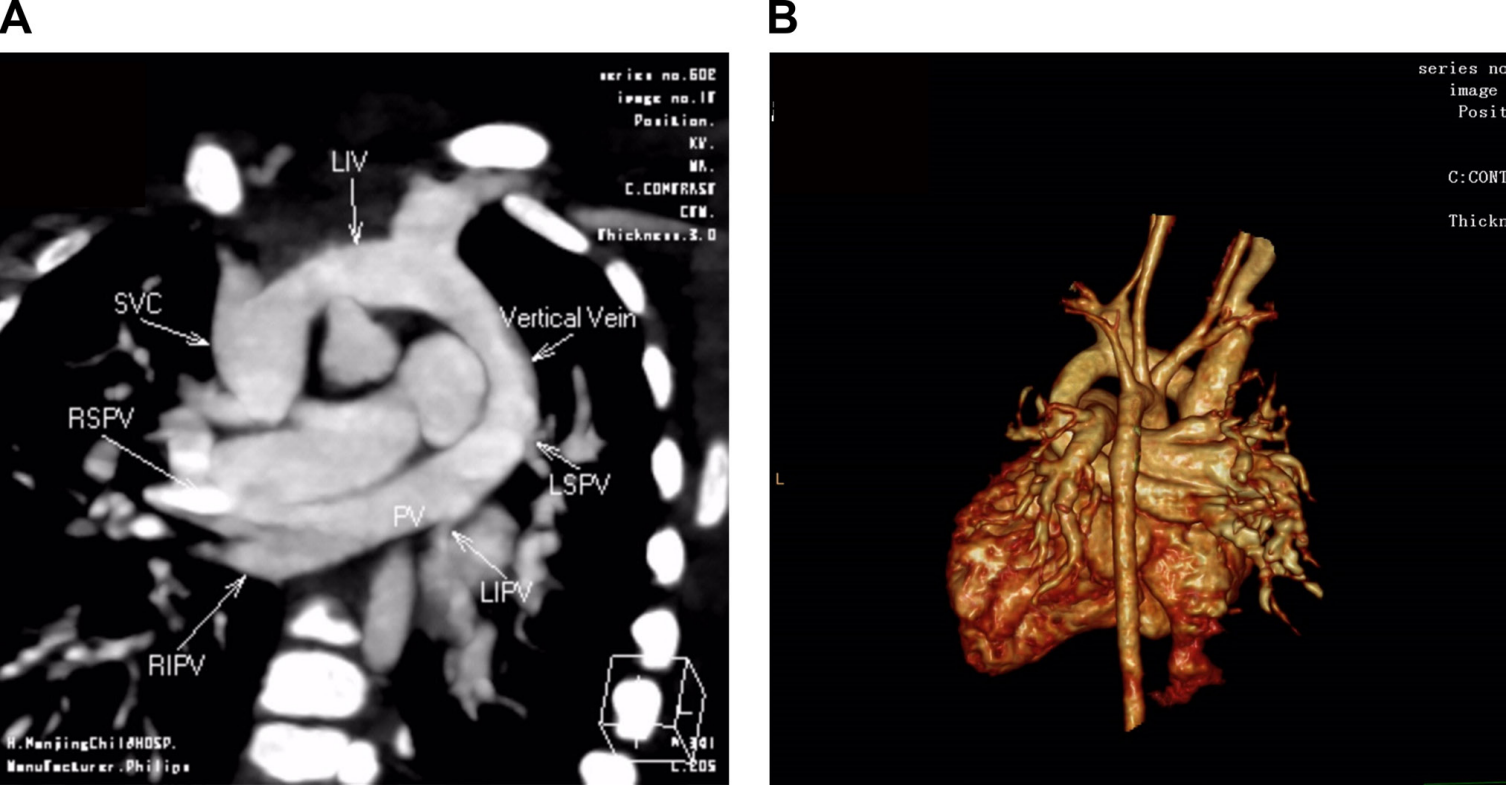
CT angiography in a 5-month-old girl with supracardiac total anomalous pulmonary venous connection (TAPVC) and unrestrictive ASD Multidetector CT angiography oblique coronal image (A) and volume rendered image (B) shows four individual pulmonary veins joining in a retrocardiac venous confluence and draining into the left innominate vein (LIV) via a vertical vein (VV). ASD: atrial septal defect; PV: pulmonary vein; SVC: superior vena cava; RIPV: right inferior pulmonary vein; RSPV: right superior pulmonary vein; LSPV: left superior pulmonary vein; LIPV: left inferior pulmonary vein; LIV: left innominate vein.
